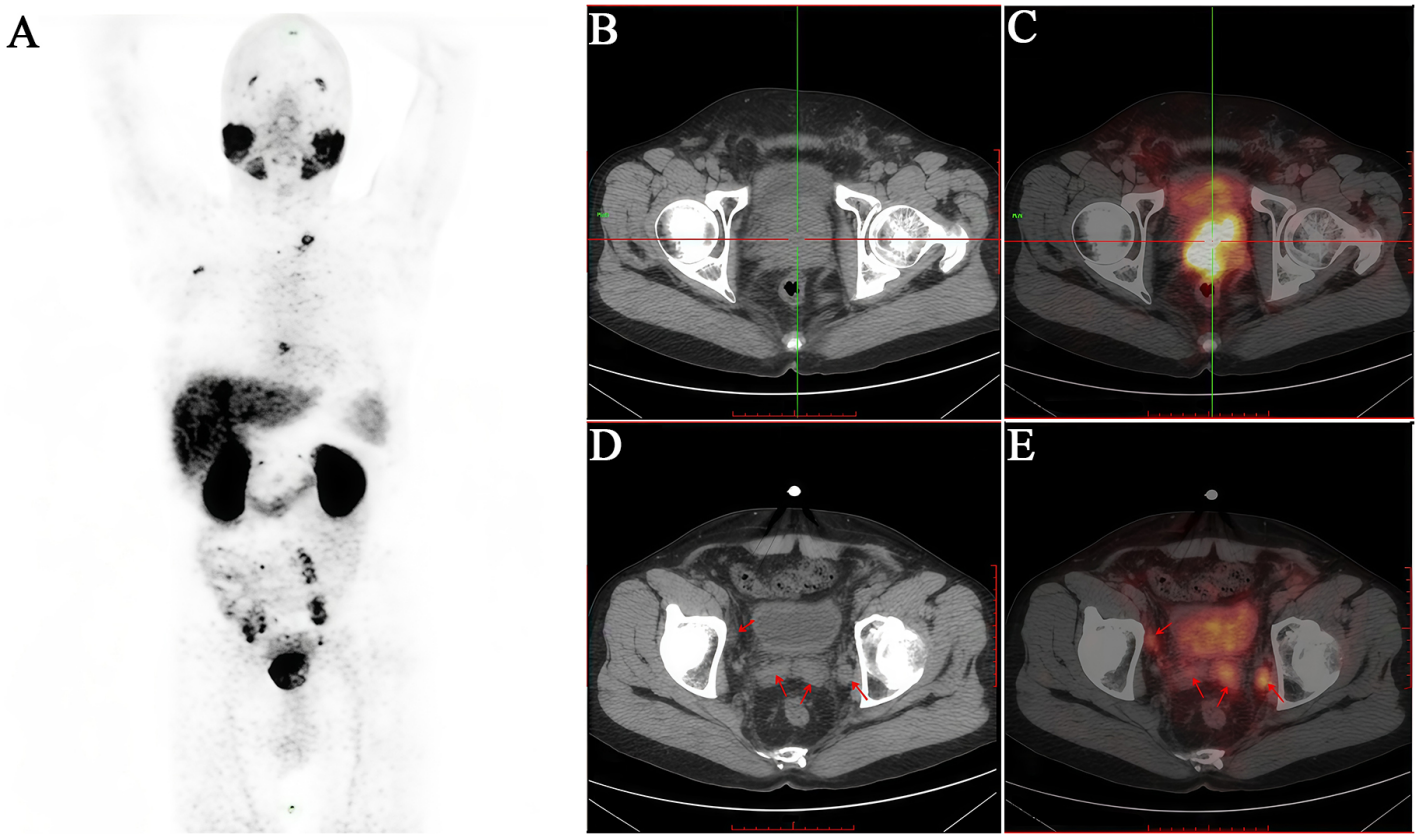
A 51-year-old male patient diagnosed with prostatic acinar adenocarcinoma (Gleason 4+4=8) by biopsy received an intravenous injection of ^18^F-PSMA. (A) PET/CT scan was performed 50 minutes later. (B, C) Multiple nodular and mass-like abnormal radioactive concentration shadows were observed in both lobes of the prostate (SUVmax 13.0). (D, E) The bilateral seminal vesicles were enlarged, especially the left side (SUVmax 3.0). They showed increased radioactive uptake. Additionally, the posterior wall of the bladder exhibited thickening with an indistinct boundary with the prostate, suggesting invasion. The patient subsequently underwent transurethral resection of bladder lesions, and the postoperative pathology was consistent with the results of the prostate biopsy.
